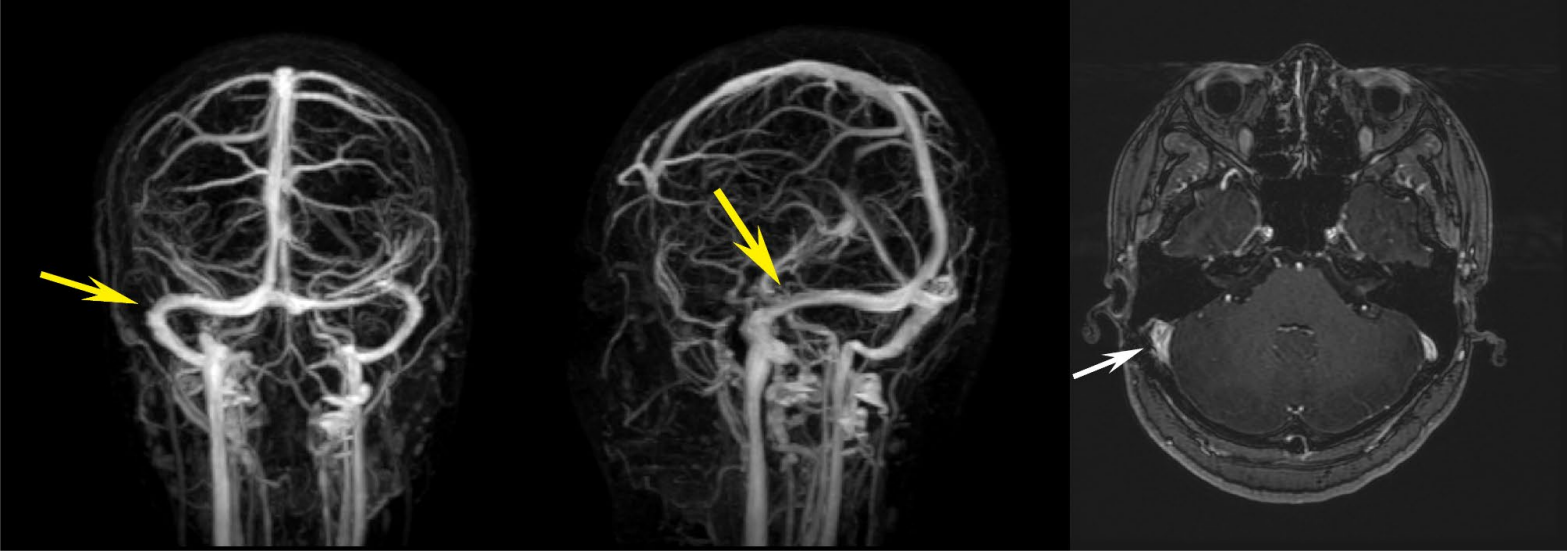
MRV (yellow arrows) and contrast-enhanced MRI (white arrow) showing resolved right sigmoid sinus stenosis after the second surgery
